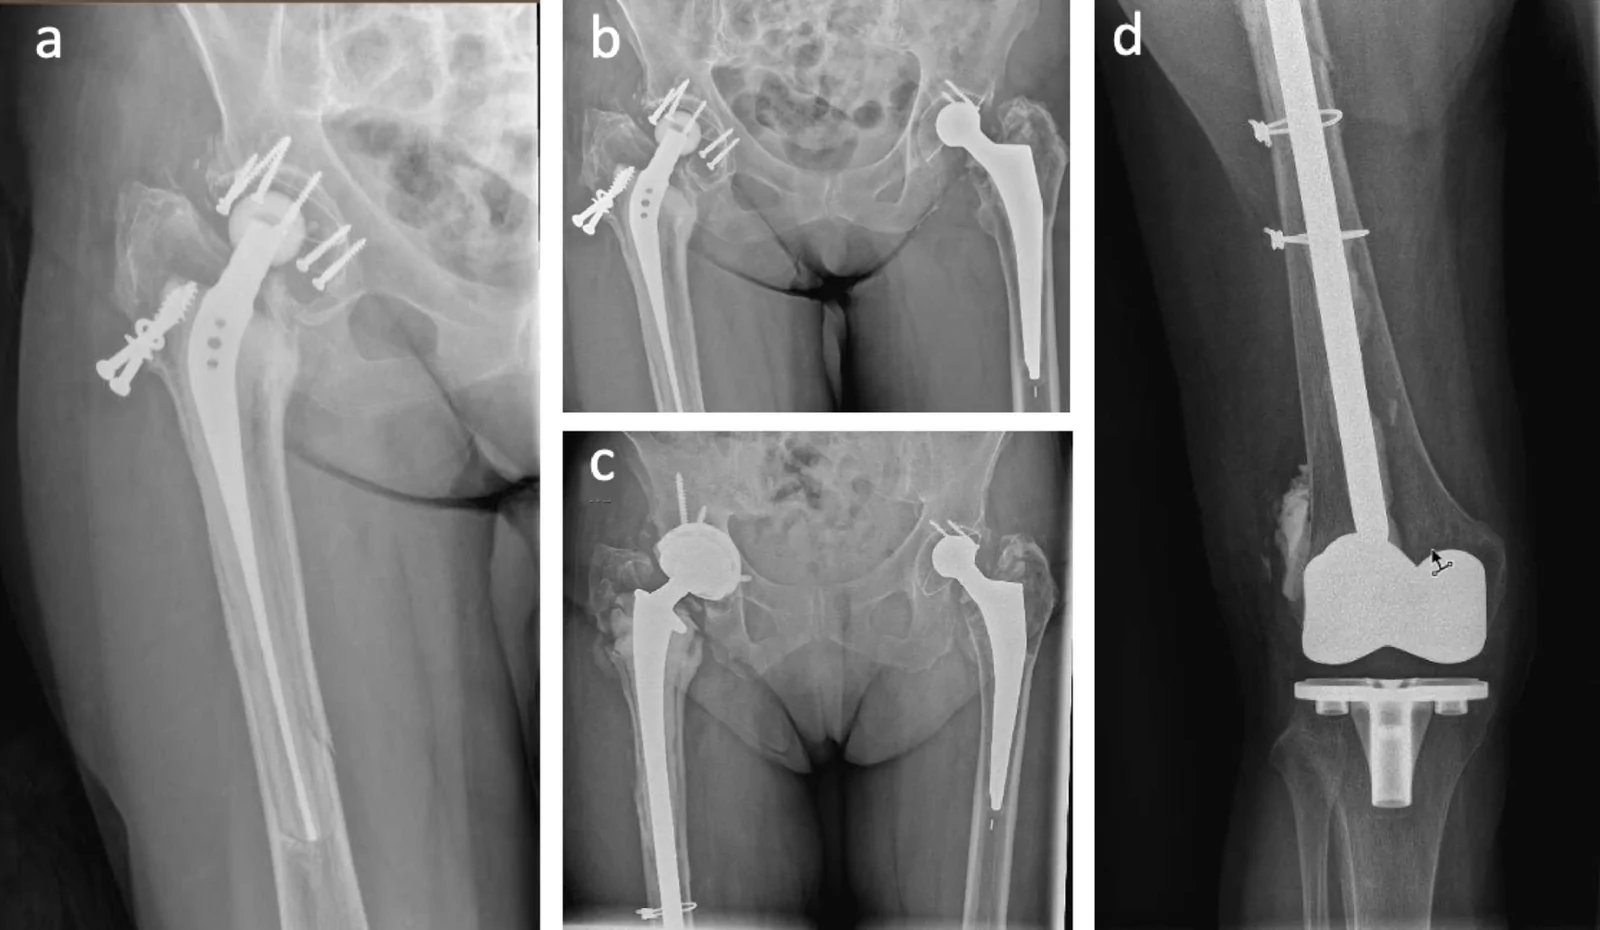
Radiographs demonstrating stabilization of a periprosthetic femoral fracture using an extended long cemented stem. Preoperative a femur and b pelvis radiographs showing a periprosthetic fracture in the setting of a structurally compromised, non-supportive diaphysis. Postoperative c pelvis and d distal femur radiographs following revision with a long cemented femoral prosthesis to bypass the diaphyseal defects and restore immediate stability
